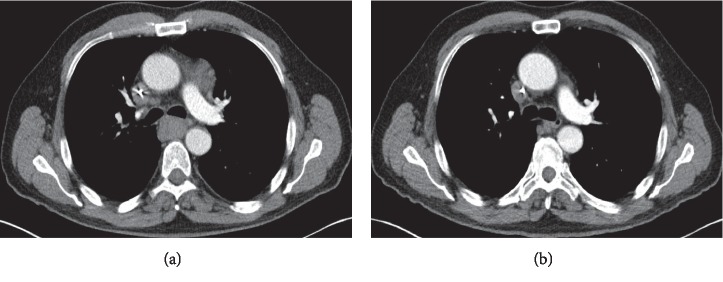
Computed tomography (CT) scan of the chest with contrast before and after nivolumab. (a) CT chest before initiation of nivolumab; an axial image depicting significant mediastinal and right hilar lymphadenopathy. (b) CT chest after 12 cycles of nivolumab; no hilar or mediastinal lymphadenopathy identified.
